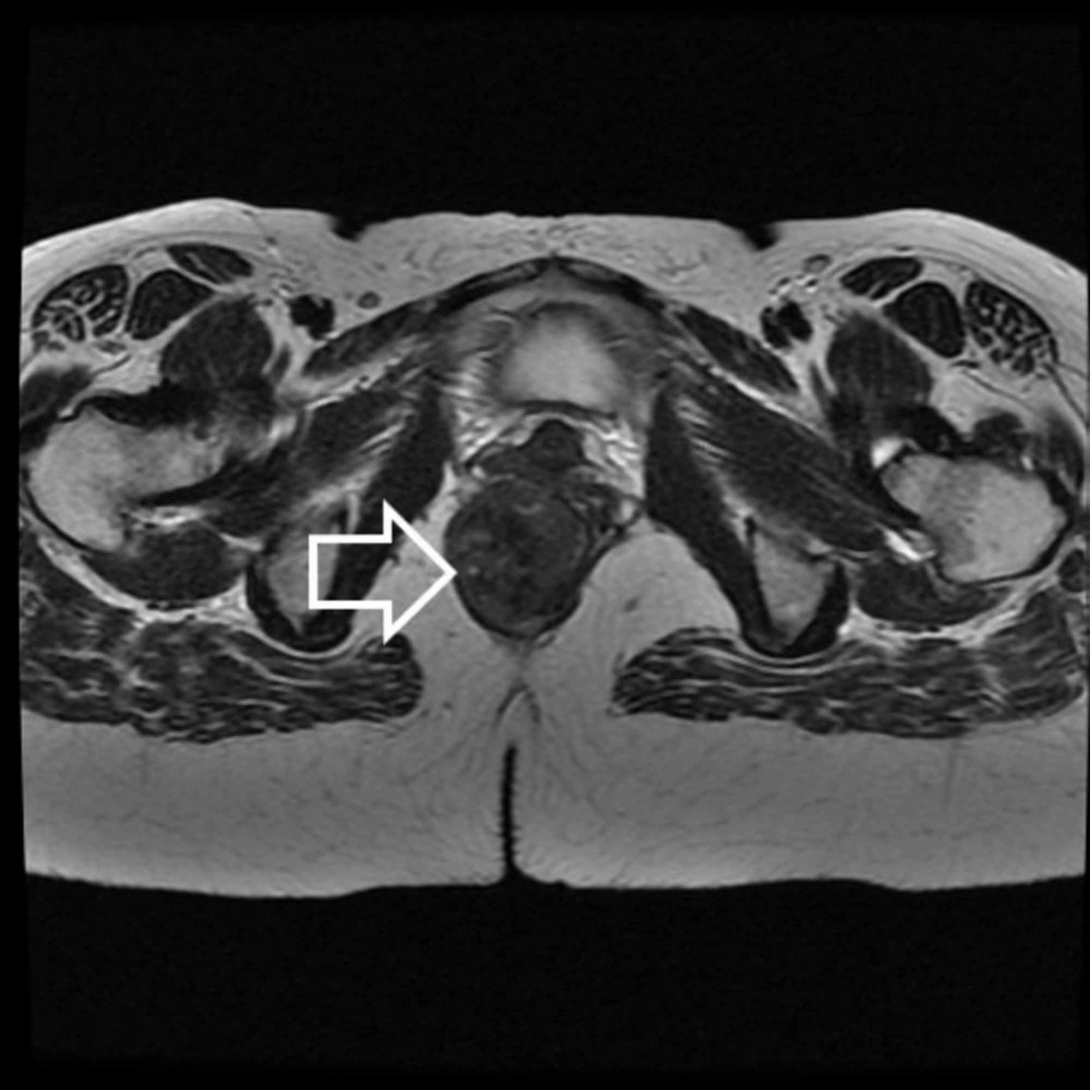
Axial T2-weighted MRI showing a mass of the right later wall of the lower rectum (white arrow)
